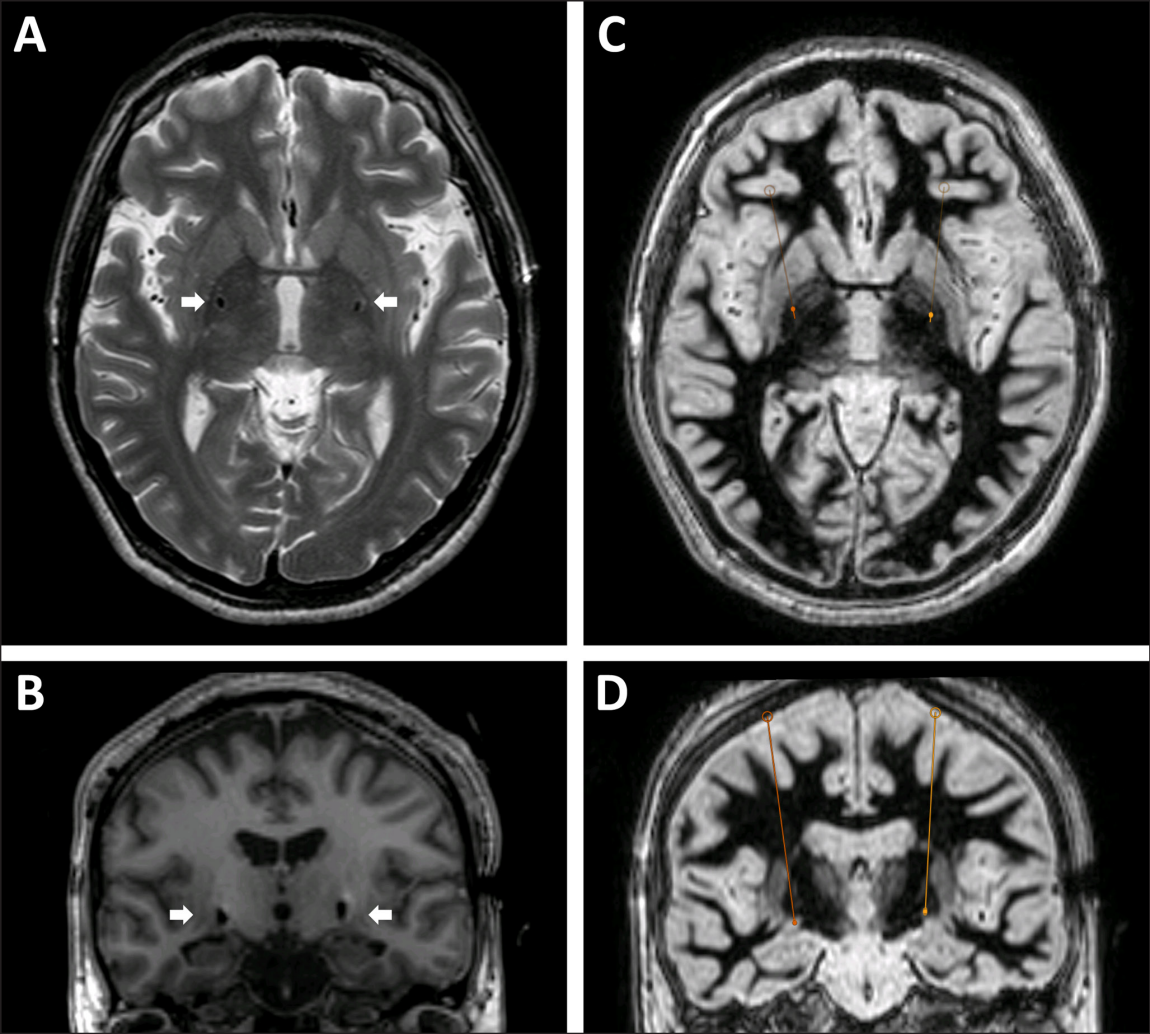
Pallidal DBS lead placement. A. Axial T2-weighted postoperative MRI image showing DBS lead artifacts (white arrows) at the AC-PC plane. B. Coronal T1-weighted posteropative MRI image showing DBS lead artifacts (white arrows) in the region of the posterior pallidum. C and D. Axial (C) and coronal (D) inversion recovery images showing borders of the globus pallidus externa and interna. DBS lead trajectories are indicated by orange lines. Intended left DBS target: 21 mm lateral, 2 mm anterior, 1 mm superior to MC with final DBS tip location extended 3.5 mm beyond the targeting plane to reach the base of the pallidum (above the optic tract). Intended right DBS target: 21.3 mm lateral, 2.3 mm anterior, 1 mm superior to MC with final DBS tip location extended 3.5 mm beyond the targeting plane. Left DBS lead location at targeting plane: 21.2 mm lateral, 1.9 mm anterior, 1 mm superior to MC (radial error of 0.3 mm). Right DBS lead location at targeting plane: 21.3 mm lateral, 2.3 mm anterior, 1 mm superior to MC (radial error of 0). MC=mid-commissural point (half point between anterior commissure to posterior commissure).
